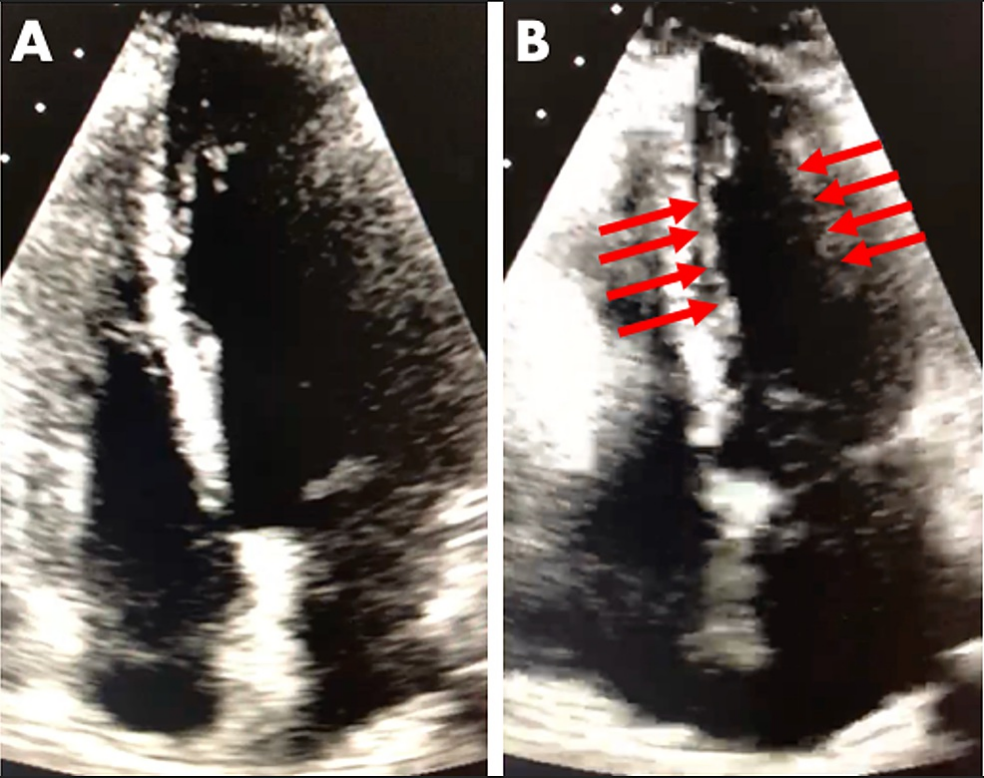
Transthoracic echocardiogram of the patient demonstrating midventricular ballooning syndrome. (A) Left ventricular diastole. (B) Severe hypokinesis of the midleft ventricular wall (red arrows) during systole.
